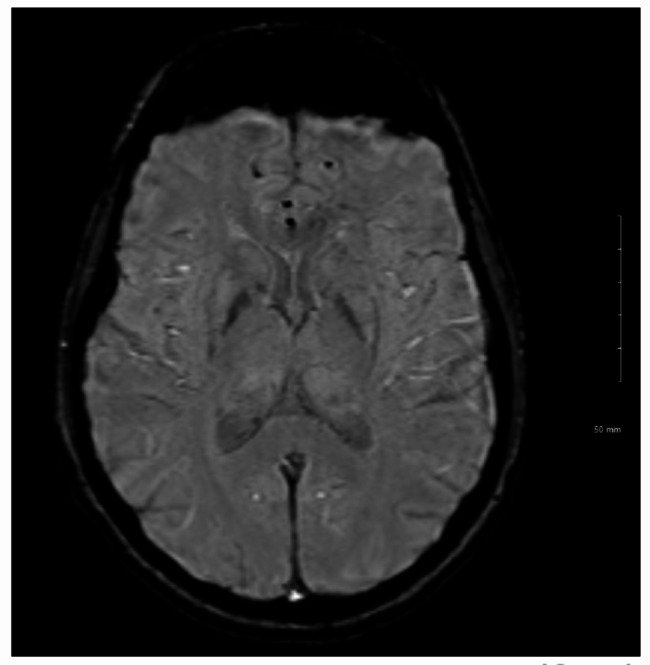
Axial susceptibility-weighted (SWI) MRI scan
